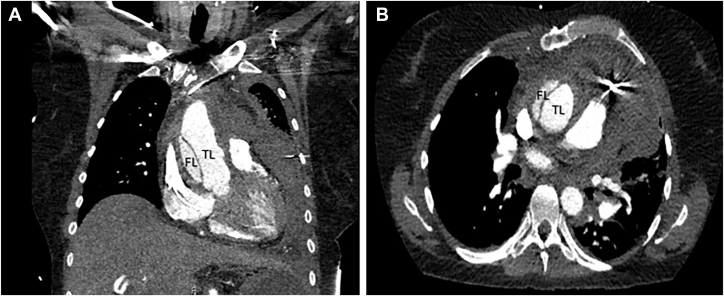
(A) A computed tomography coronal image showing the type A aortic dissection confined to the donor aorta. (B) A computed tomography transversal image showing the type A aortic dissection confined to the donor aorta. (FL, false lumen; TL, true lumen.)
